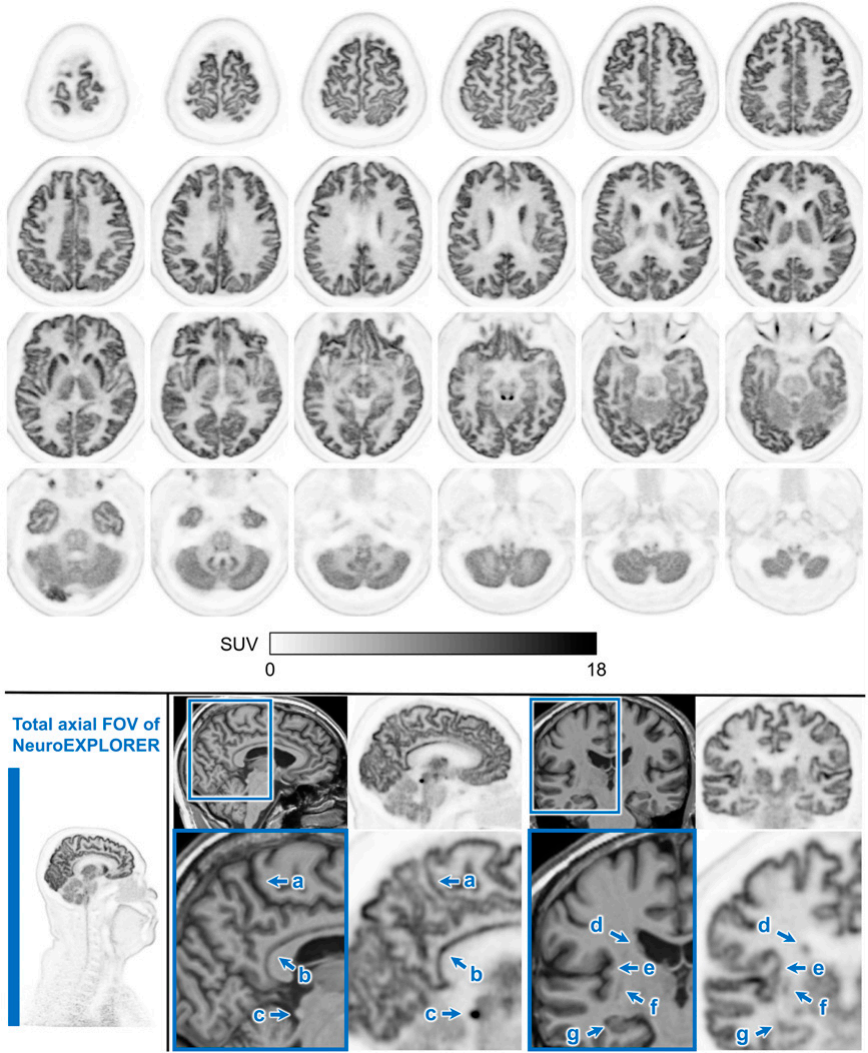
^18^F-FDG PET image (40–70 min after injection) on NX. (Top) Top-to-bottom axial slices showing excellent spatial resolution of NX, which provides clear delineation of cortical ribbon along with subcortical nuclei. (Bottom, far left) Sagittal image showing long AFOV of NX (49.5 cm), which allows inclusion of neck and upper chest structures. (Bottom right) Two pairs of T1-weighted MRI (left) and NX (right) image slices. Beneath are zoomed-in regions demonstrating NX’s high spatial resolution for cortical ribbon structures and small brain nuclei, including marginal sulcus (a), posterior cingulate cortex (b), inferior colliculus (c), caudate (d), insular cortex (e), putamen (f), and hippocampus (g).
